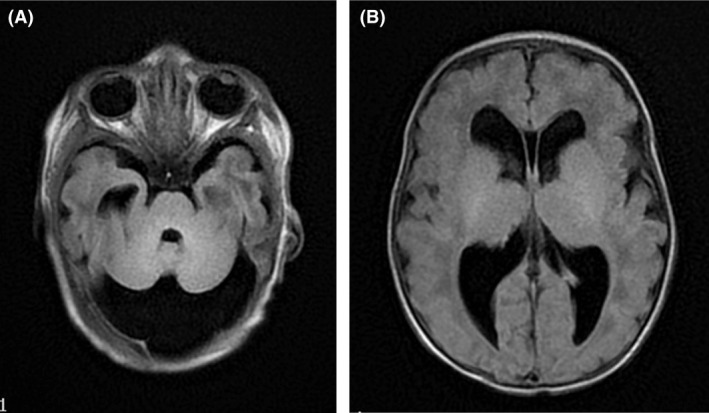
(A) Cranial MRI shows cerebellar hypoplasia. (B) Cranial MRI shows bilateral ventriculomegaly.
